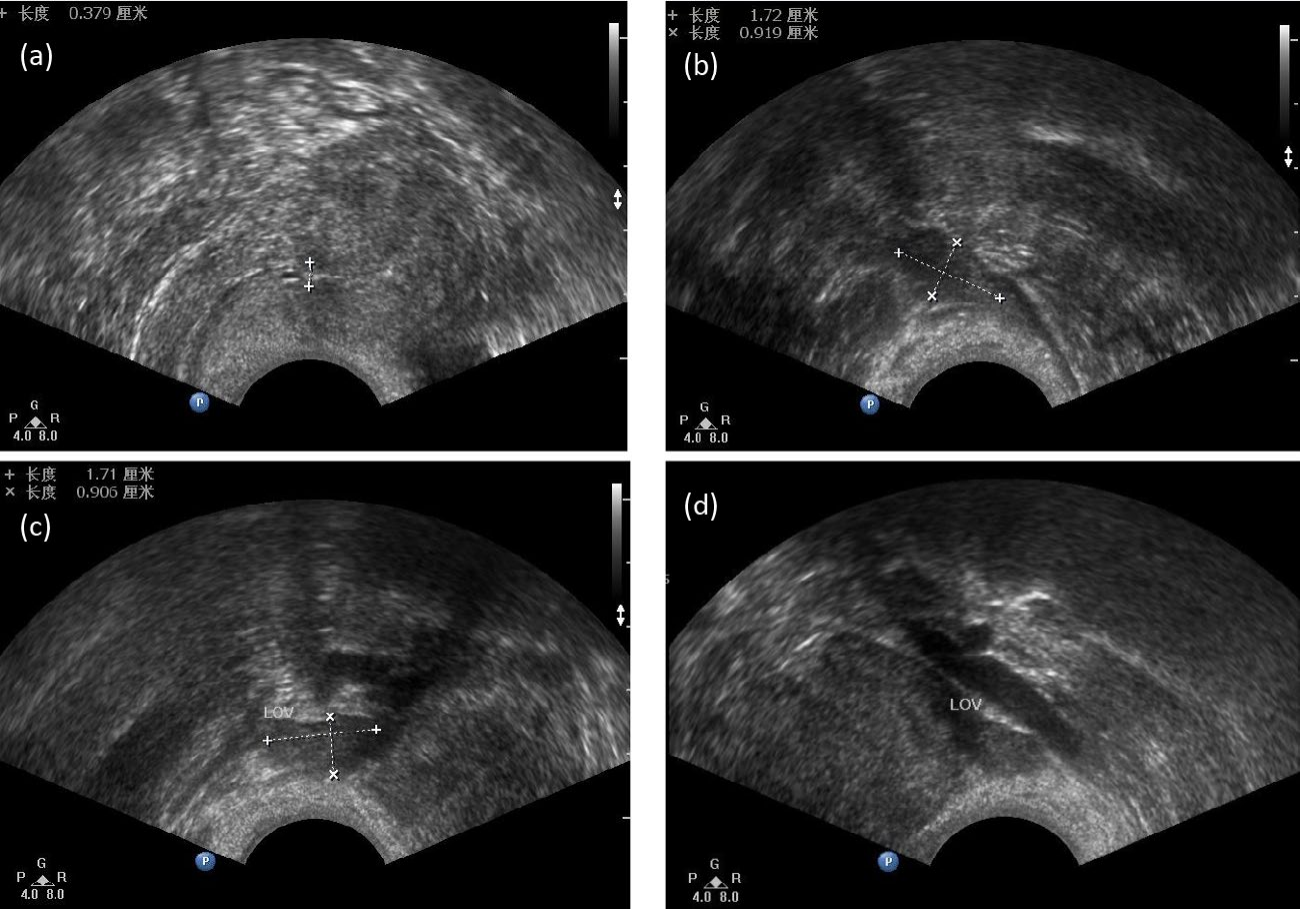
Transvaginal ultrasound image of the POI subject. (a) The thickness of endometrium. (b) Size of the right ovary. (c and d) Size of the left ovary
